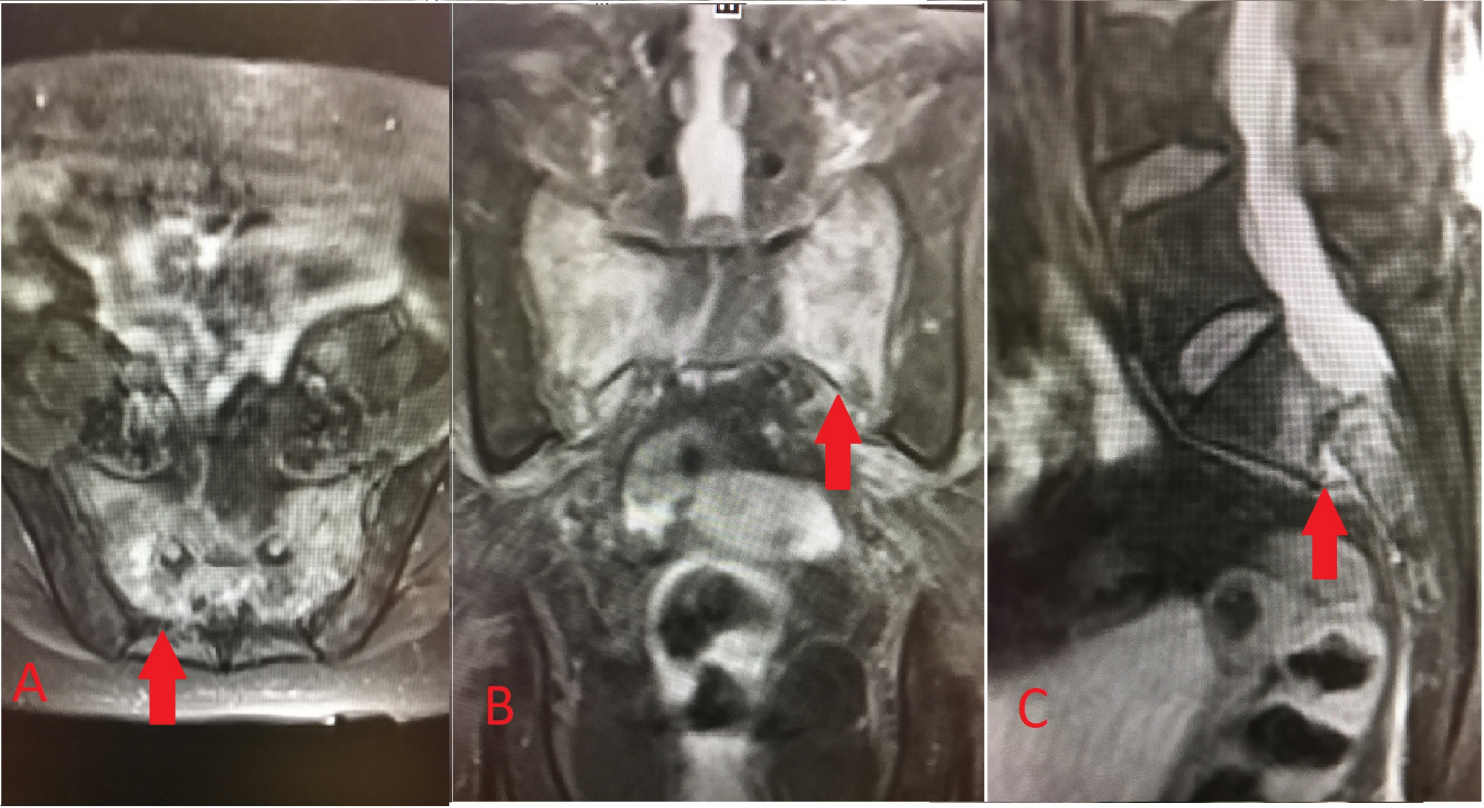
MRI of the sacrum and pelvis A. Axial T2-weighted (T2W) short tau inversion recovery (STIR) sequence demonstrating hyperintensity (arrow) located within the bilateral sacral ala and the body of the sacrum; B. Coronal T2W STIR; C. Sagittal T2W STIR again demonstrating hyperintensity but also showing  S1-S2 anterolisthesis (arrow)
